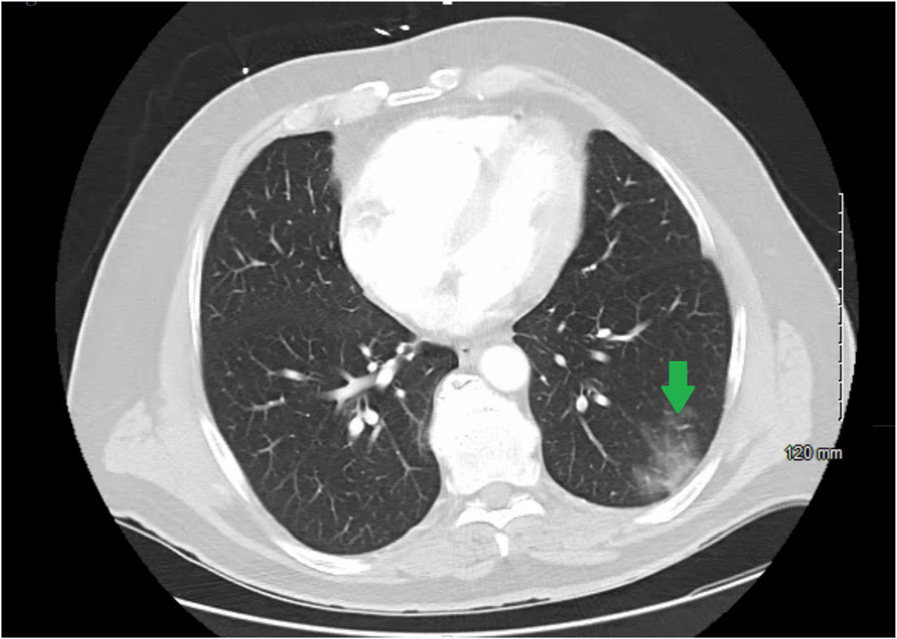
Computed tomography (CT) angiogram of the chest (lung window) showing an evolving small, left lower lobe, pulmonary infarct (green arrow)
